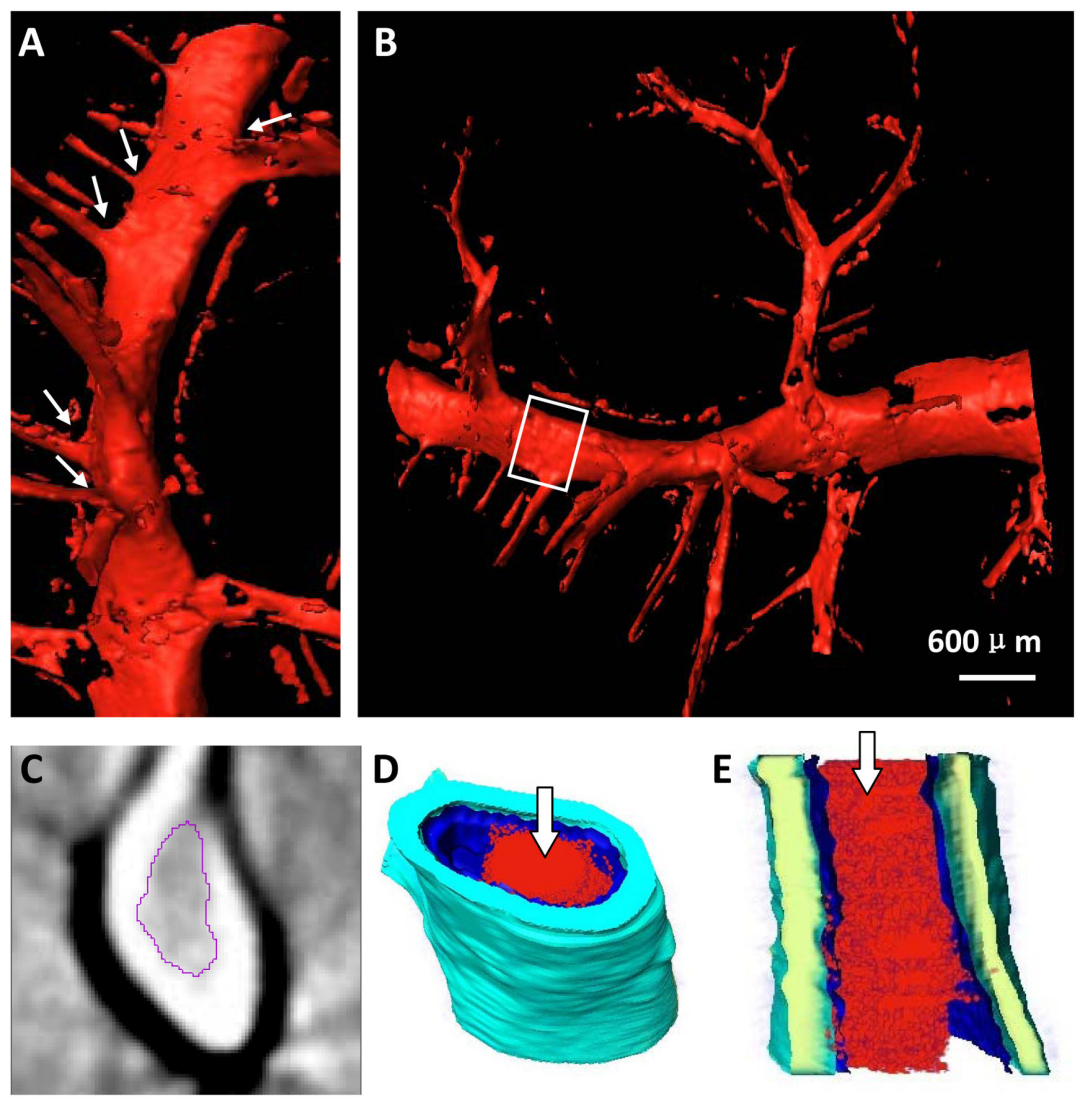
The two profiles of the 3D reconstruction are shown in (A) and (B). Obvious distortion deformation is presented in (A), and the vessel branch angles, as indicated by the arrows, appear stiffer. (C) One CT slice and (D) the vessel segment, shown in the white rectangle region in (B), are presented. The thrombus inside the vessel in (C) is segmented. (E) Longitudinal section of the vessel segment in (D). The inner red part indicated by the arrows in (D) and (E) is a thrombus.
